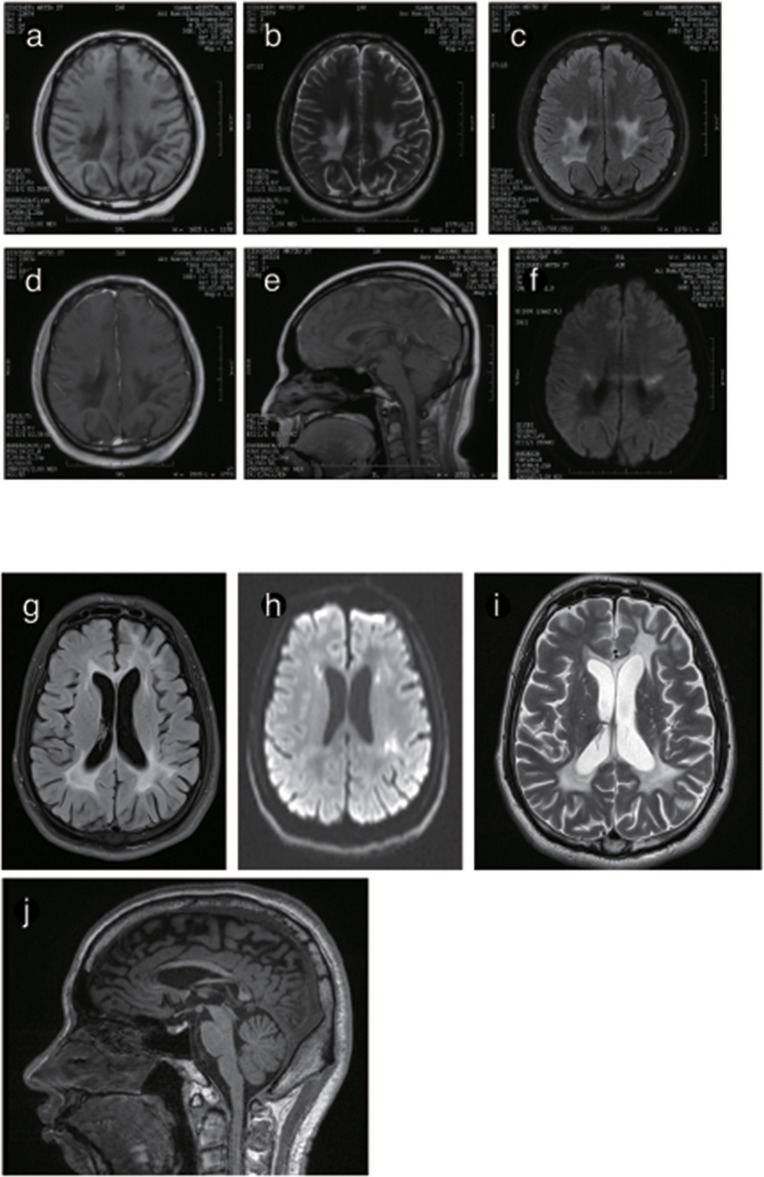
Representative images from patient P4 and P5's brain MRI. (a) Axial MRI shows decreased signal intensity on Tl-weighted images, increased signal intensity on T2-weighted images (b) and a predominant FLARE signal in periventricular areas with no enhancement (c) or ring-like enhancement after contrast injection (d). (e) The sagittal T1-weighted image shows multiple hypointense lesions in the postural area of the corpus callosum. (f) The ADC image shows a periventricular diffusion restricted signal. (g) Axis FLARE image shows asymmetrical patchy lesions and inhomogeneous cerebral white matter abnormalities in the regions around the lateral ventricle (h). The posterior areas of the periventricular white matter show restricted diffusion (i). (j) The sagittal T1-weighted image shows that the anterior and posterior segments of the corpus callosum are affected.
